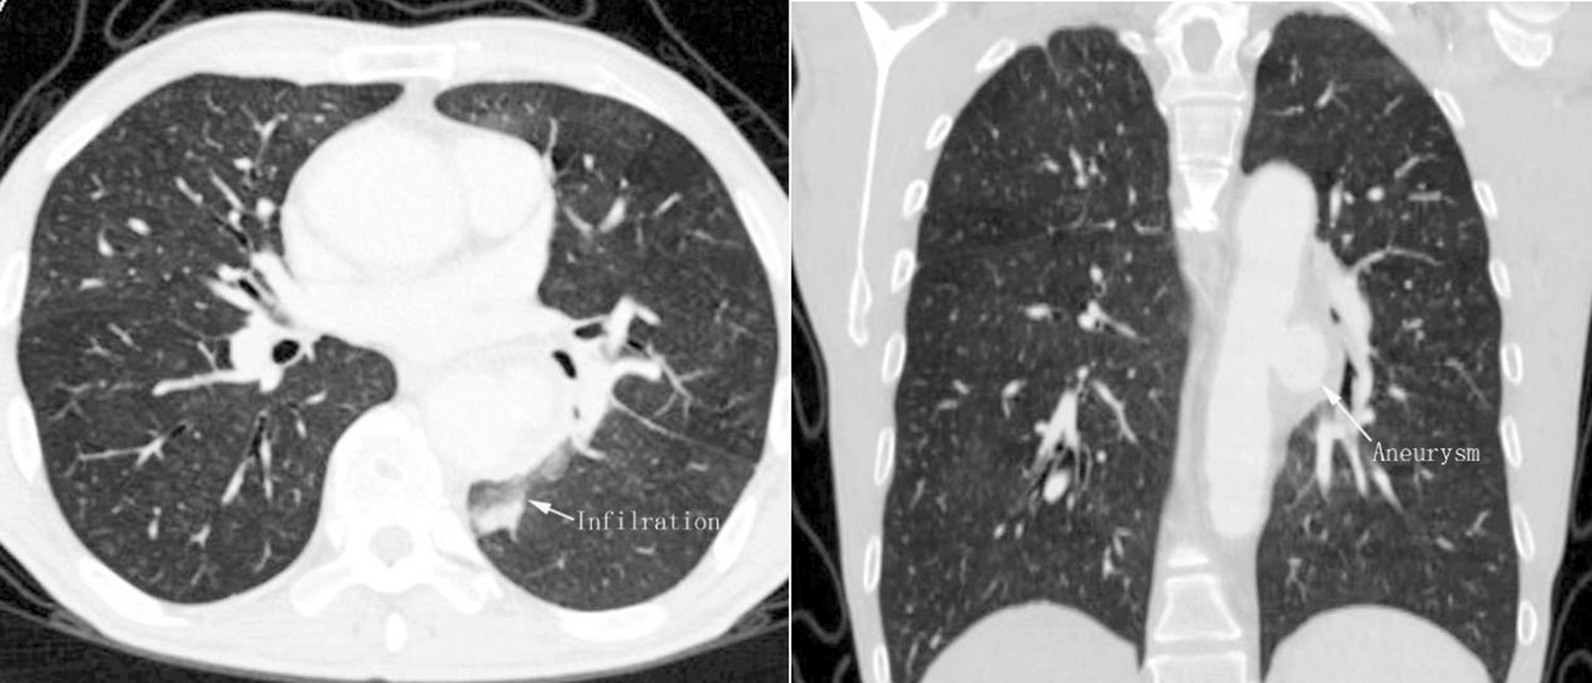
(Left), (right): Axially and coronally reconstructed CT images revealed a 21 × 30 mm saccular aneurysm with rupture into the superior segment of left lung lower lobe
